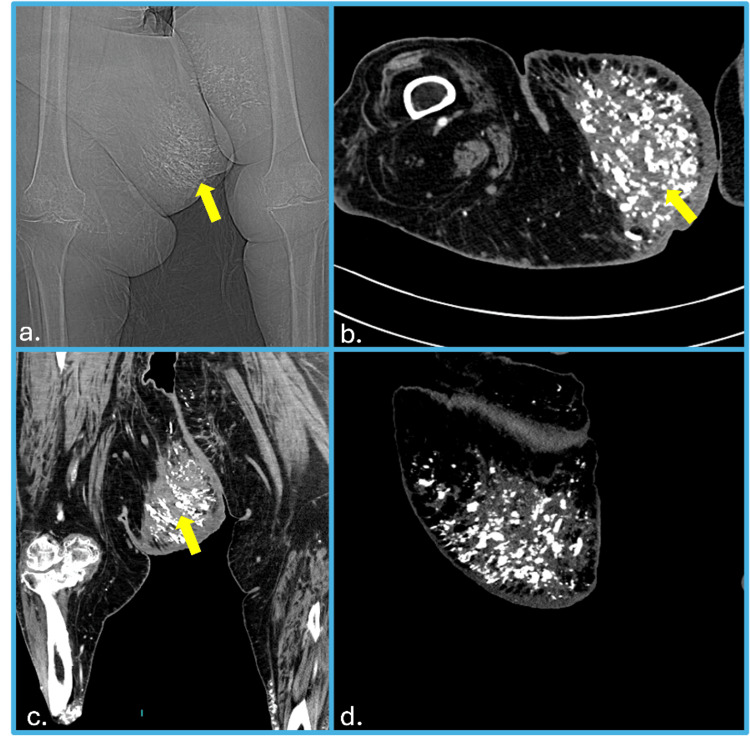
CT imaging of the right lower extremity. (a) Topogram of the lesion with the yellow arrow pointing to the mass grossly seen on the right medial thigh. (b) Axial soft-tissue view of the right lower extremity with the yellow arrow pointing to the mass. (c) Coronal soft-tissue view of the right lower extremity with the yellow arrow pointing to the mass. (d) A sagittal soft-tissue view of the right lower extremity mass.
